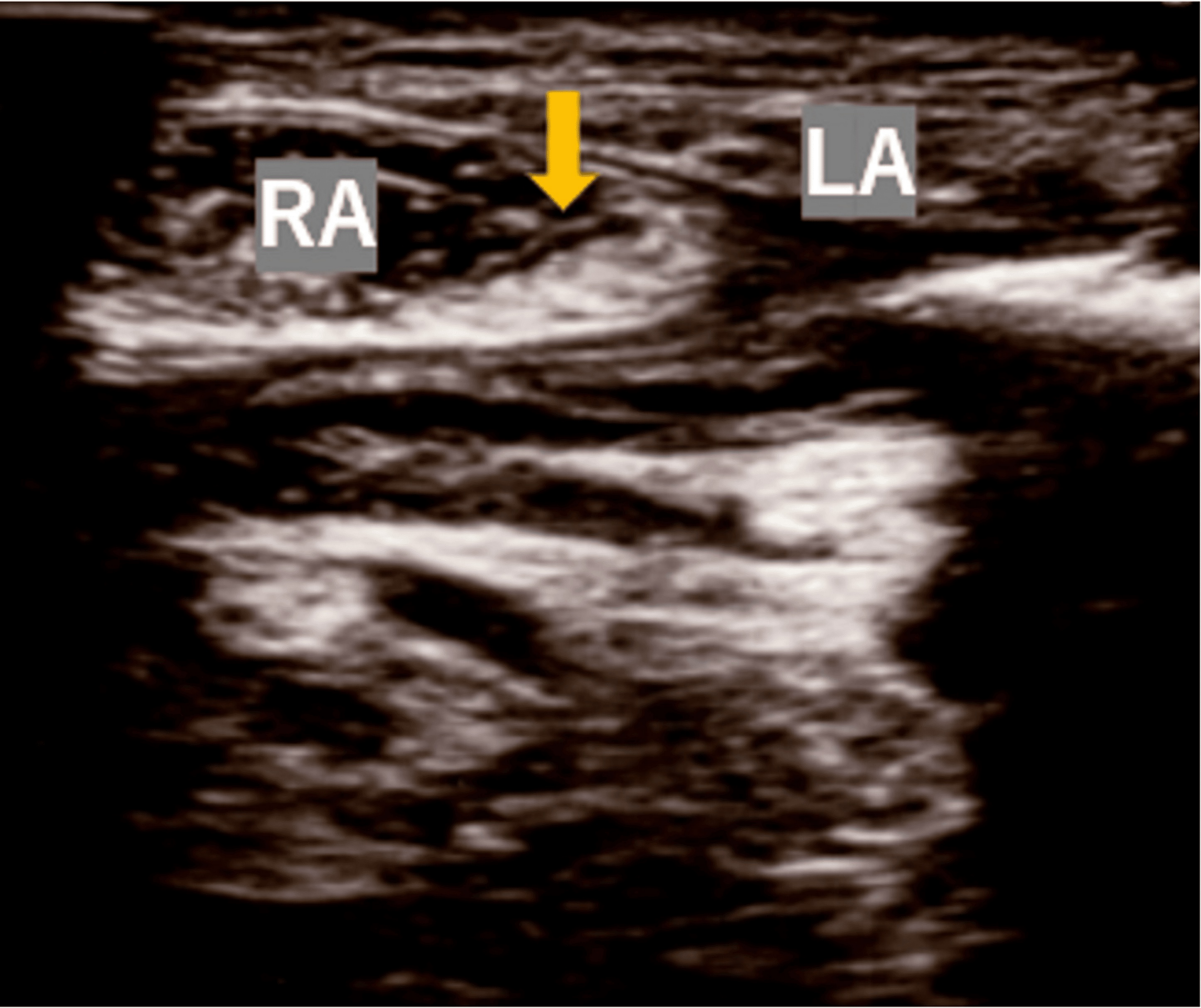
Ultrasound image of the trigger point at the RA muscle on the second visit to our pain clinic. A hyperechoic area (arrow) was identified at the inner edge of the RA muscle LA: linea alba; RA: rectus abdominis
